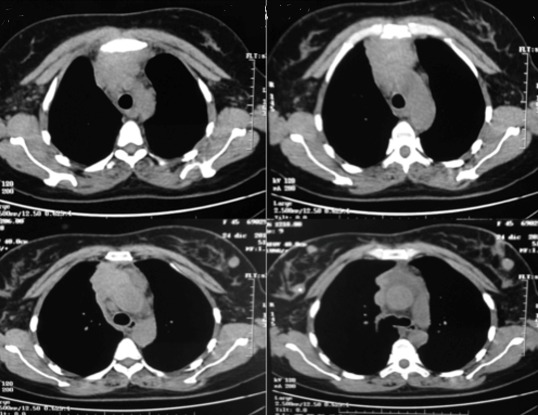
Chest CT: solid anterior mediastinal mass measuring 50 x 40 x 35mm in intimate contact with mediastinal vascular structures without cleavage plane and sternal commitment
